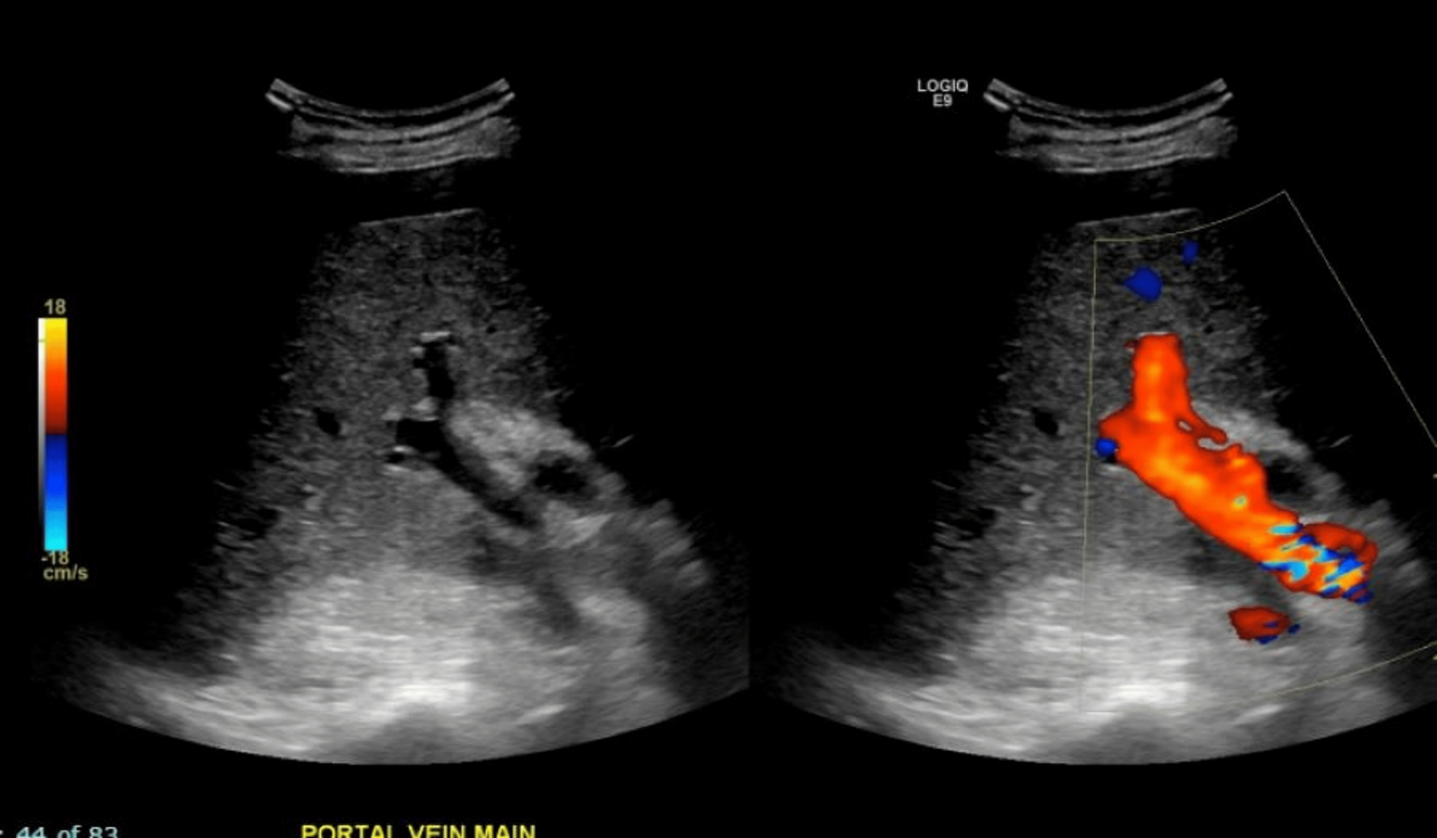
Right upper quadrant ultrasound demonstrating nodular liver contour, patent main portal vein, and absence of cholelithiasis or definite masses
